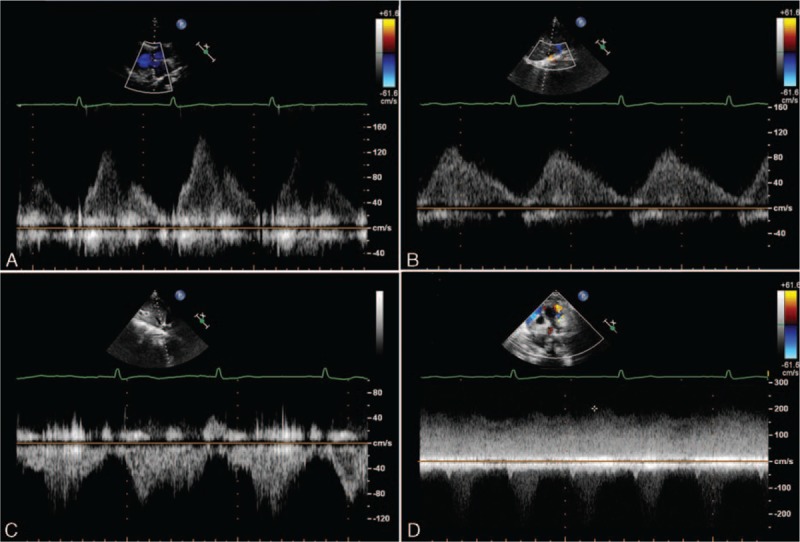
Pulse Doppler of different patterns in pulmonary veins, anomalous pulmonary veins (APVs), obstructed APVs, and systemic veins. (A) Pulse Doppler of phasic flow in the left pulmonary vein, varying with the cardiac cycle. (B) Pulse Doppler of phasic flow in the APV, varying with the cardiac cycle. (C) Pulse Doppler of nonphasic flow in the inferior vena cava with low velocity. (D) Pulse Doppler of APV with an obstruction, nonphasic flow with high velocity.
